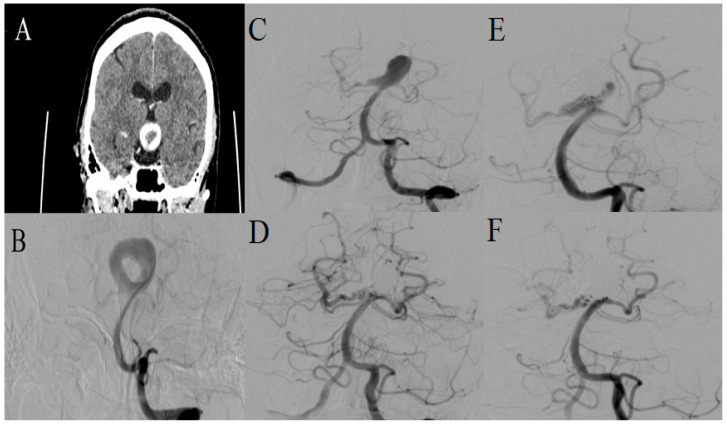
(A) Contrast-enhanced CT identified a ring-shaped wide-necked aneurysm at the basilar tip; (B) DSA of the posterior circulation displaying a giant partially thrombosed donut-shaped aneurysm at the basilar tip. (C) Preprocedural DSA following a treatment delay demonstrated significant thrombosis within the majority of the aneurysm; (D) Postprocedural DSA after placement of the stent over aneurysm neck and subsequent coiling; (E) DSA on 6-month follow-up revealed coil impaction within the thrombus with residual aneurysm presence; (F) Postprocedural DSA following the coiling procedure.
